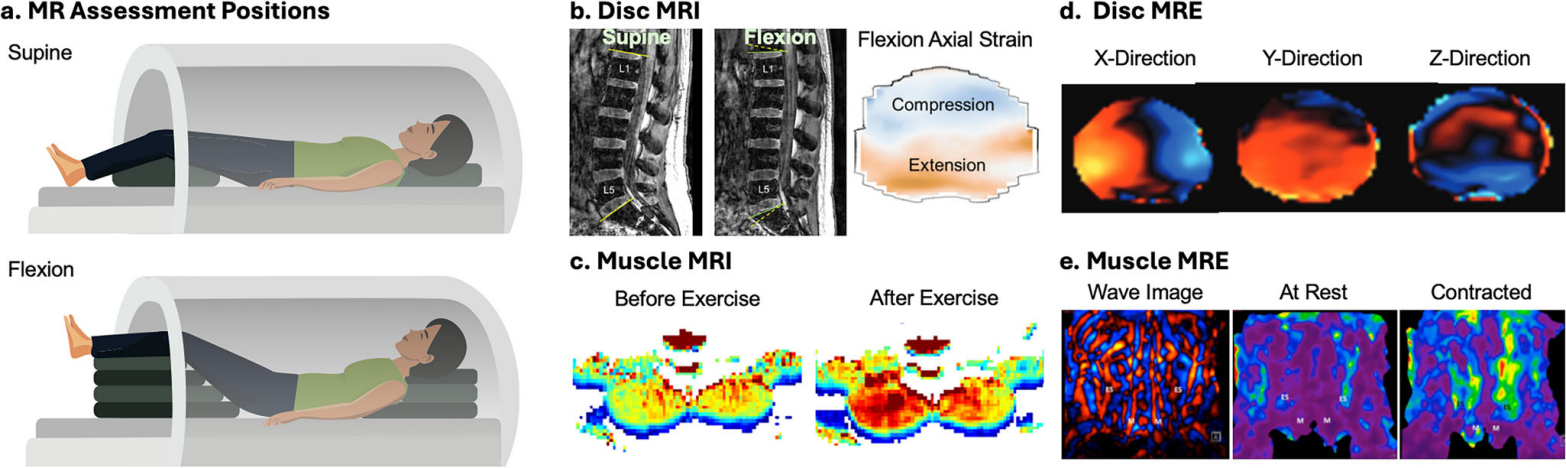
Magnetic resonance imaging (MRI) and Magnetic Resonance Elastography (MRE). MRI acquired under a different positions can be used to measure disc deformation and strain; and MRI before and after exercise can be used to estimate muscle activation through area and signal intensity change. b MRI of lumbar spine shown as midsagittal section in supine and flexion. Images are registered to calculate the axial strain in flexion relative to supine, shown in an axial section, adapted from ref. ^156^. c MRI T2 map (related to hydration) of paraspinal muscles before and after exercise showing increased T2 values after exercise, due to blood perfusion into the muscle, adapted from ref. ^239^. MRE extends MRI to provide mechanical properties rather than just anatomical information. Additional technology to induce vibration or shear waves are required (not shown). d In-plane and through-plane phase encoding MRE showing wave propagation through the disc, reproduced from ref. ^206^. e MRE of L2-L5 coronal view through the center of the muscles’ cross-section, showing the wave image and the shear modulus map while at rest and while contracting, where warmer colors indicate stiffer tissues; erector spinae (ES) and multifidus (M) both become stiffer with contraction, adapted from ref. ^225^.
